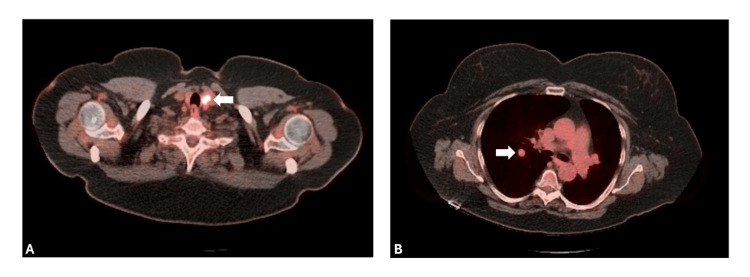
Positron emission tomography/computed tomography imaging of the neck and chest (A) A 1.2 cm left thyroid mass demonstrating high 18F-fluorodeoxyglucose (FDG) avidity (SUV 9) (white arrow). (B) Incidental finding on the same diagnostic scan demonstrating a 1.2 cm right perihilar lung nodule with mild FDG avidity (SUV 3.5) (white arrow).
